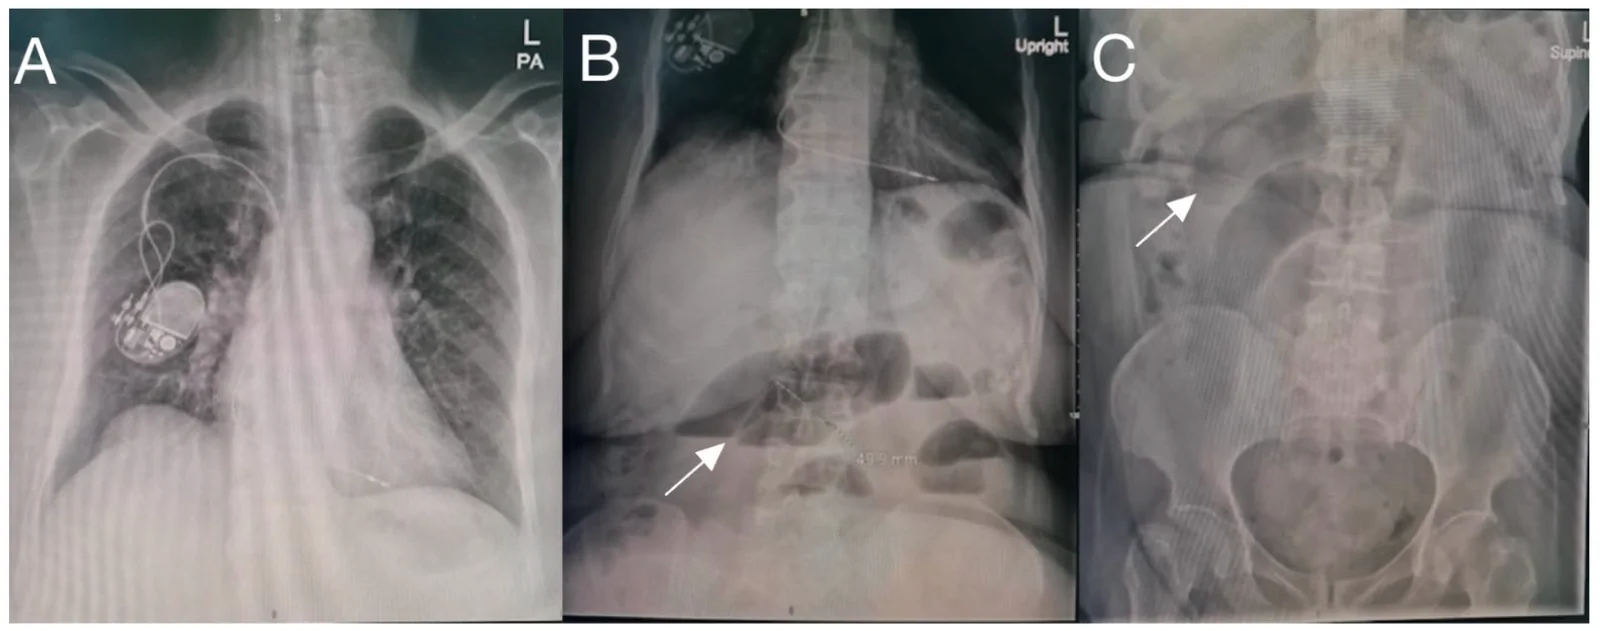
Plain X-ray: (A) PA chest X-ray showing an unremarkable study with no evidence of air under the diaphragm. (B,C) Abdominal X-ray showing prominent small bowel loops (arrow), suggestive of a potential obstruction.
